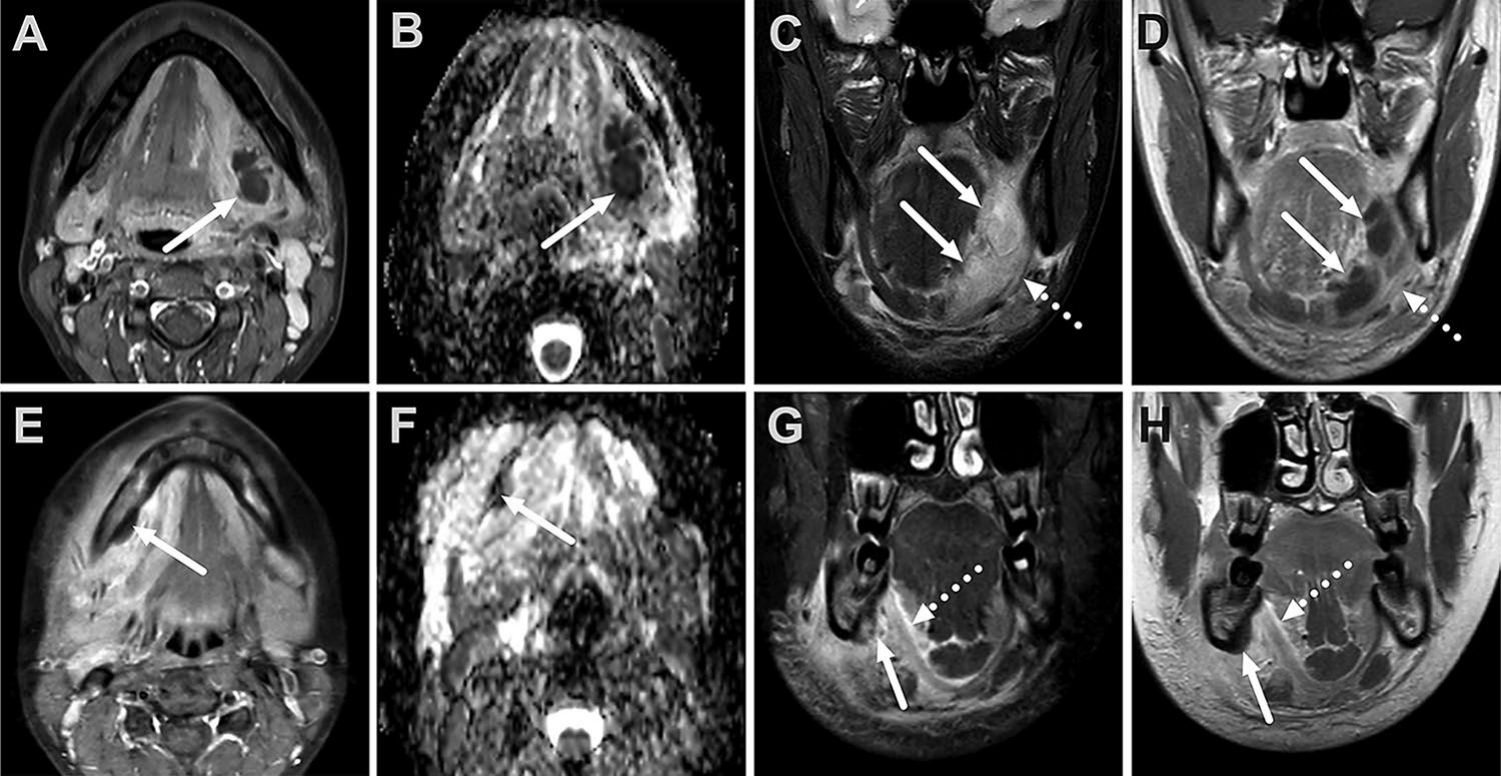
Sublingual space abscess (solid arrows) in a 25-year-old female following teeth extraction (dd. 28,38) and subsequent infection (upper row). Small submandibular space abscess (solid arrows) subperiosteally in a 13-year-old male with d47 periapical infection (lower row). T1 fat-suppressed axial images after gadolinium (A and E), showing the nonenhancing abscess core surrounded by an enhancing rim. The abscess shows restricted diffusion in the ADC map (B and F). Coronal T2 fat-suppressed and T1 post-contrast Dixon images show the hyperintense and nonenhancing abscess and its relation to the mylohyoid muscle (dotted arrows) in the sublingual space (C and D) and submandibular space (G and H)
